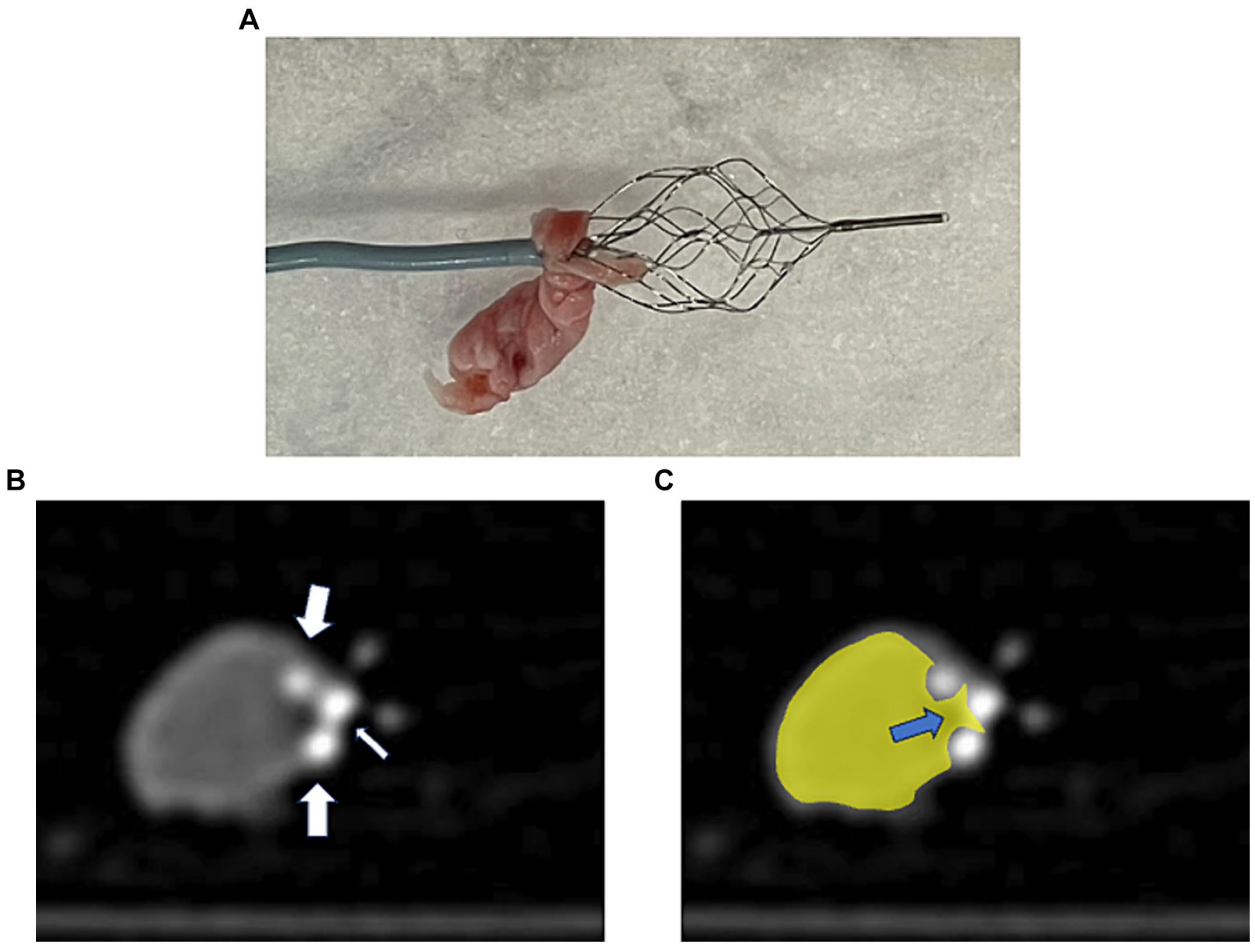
A case of thrombectomy with the PREMIER technique. (A) Actual fibrin-rich hard clot retrieved with the PREMIER technique. (B) Plain computed tomography image of fibrin-rich hard clot and EmboTrap III following the PREMIER technique. The big arrows point to the outer cage and small arrow points to the inner channel of EmboTrap III. (C) Colored version of the figure (B). The hard clot is colored yellow. The blue arrows indicate the section where the clot is pinched between the inner channel and the outer cage of EmboTrap III.
